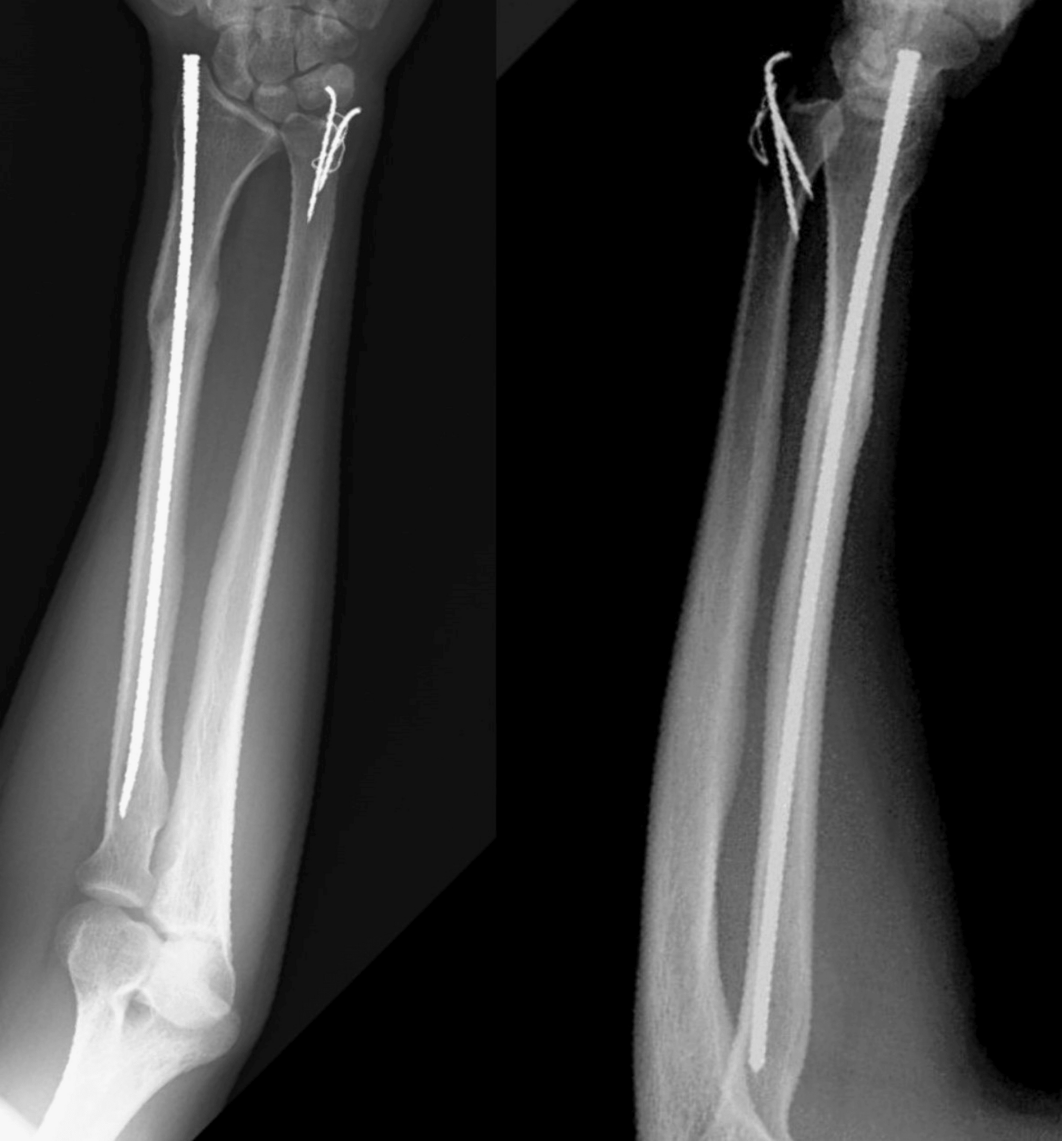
Postoperative X-ray of fracture shaft radius managed with intramedullary screw nail (one year follow-up) with TBW done for ulnar styloid fracture. TBW:  tension band wiring
